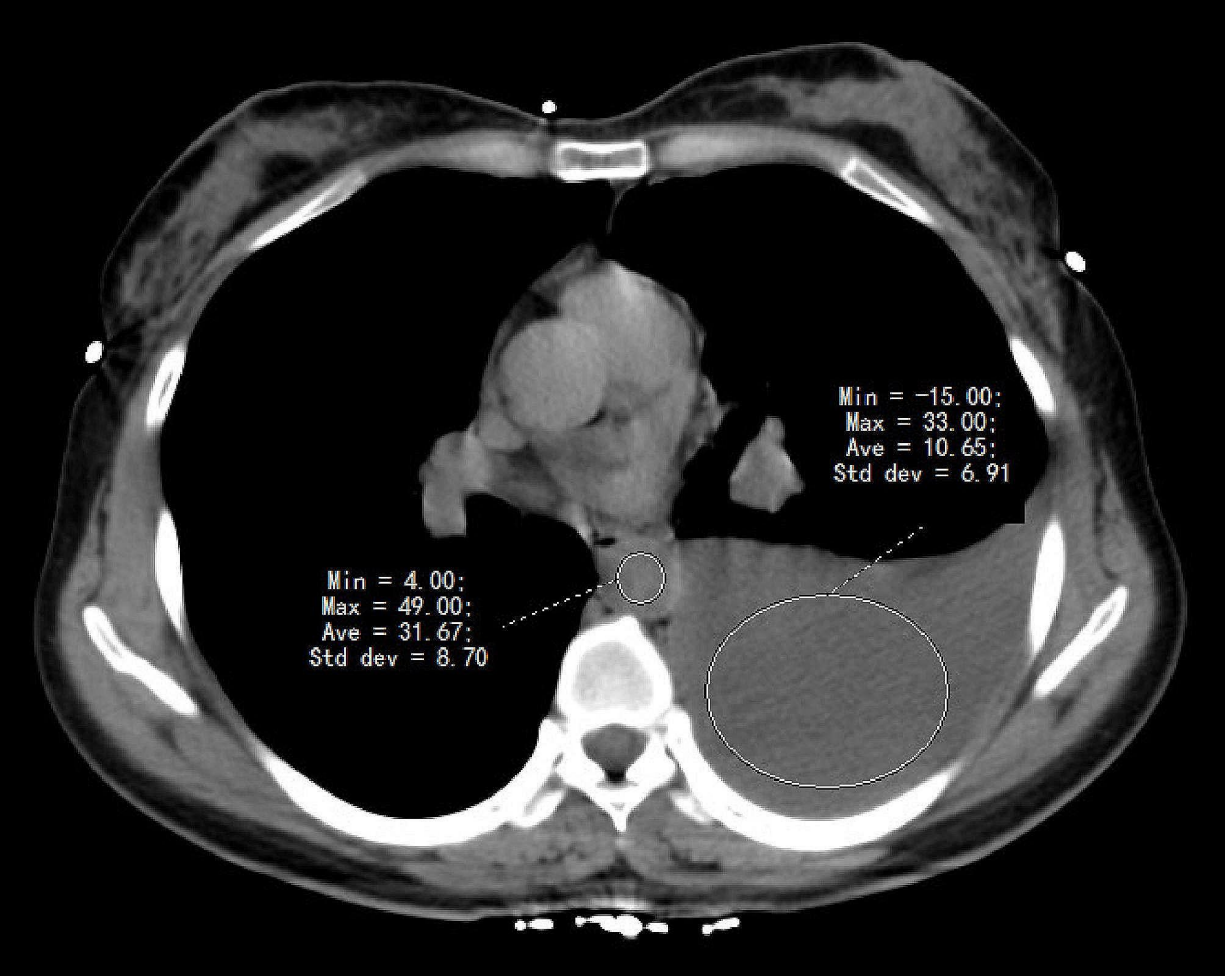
ROIs were placed on the greatest amount of effusion for the measurement of CT values. Blood pool HU values of aorta cavity were also obtained in the same manner. ROIs, regions of interest
